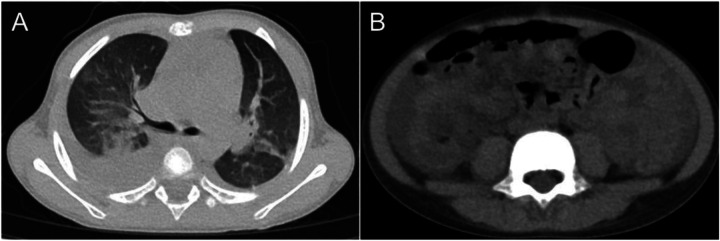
CT imaging of the chest and abdomen: (A) nodules and interstitial pneumonia observed in both lungs. (B) shadows in the perirectal region and abdominal-pelvic fat space, along with peritoneal thickening.
